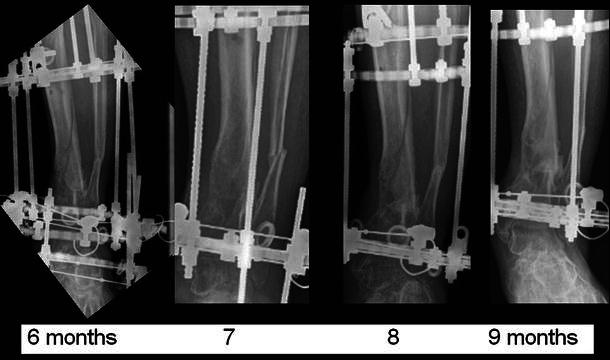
Sequential AP radiographs of the distal tibia with the frame in situ showing progressive fracture consolidation
